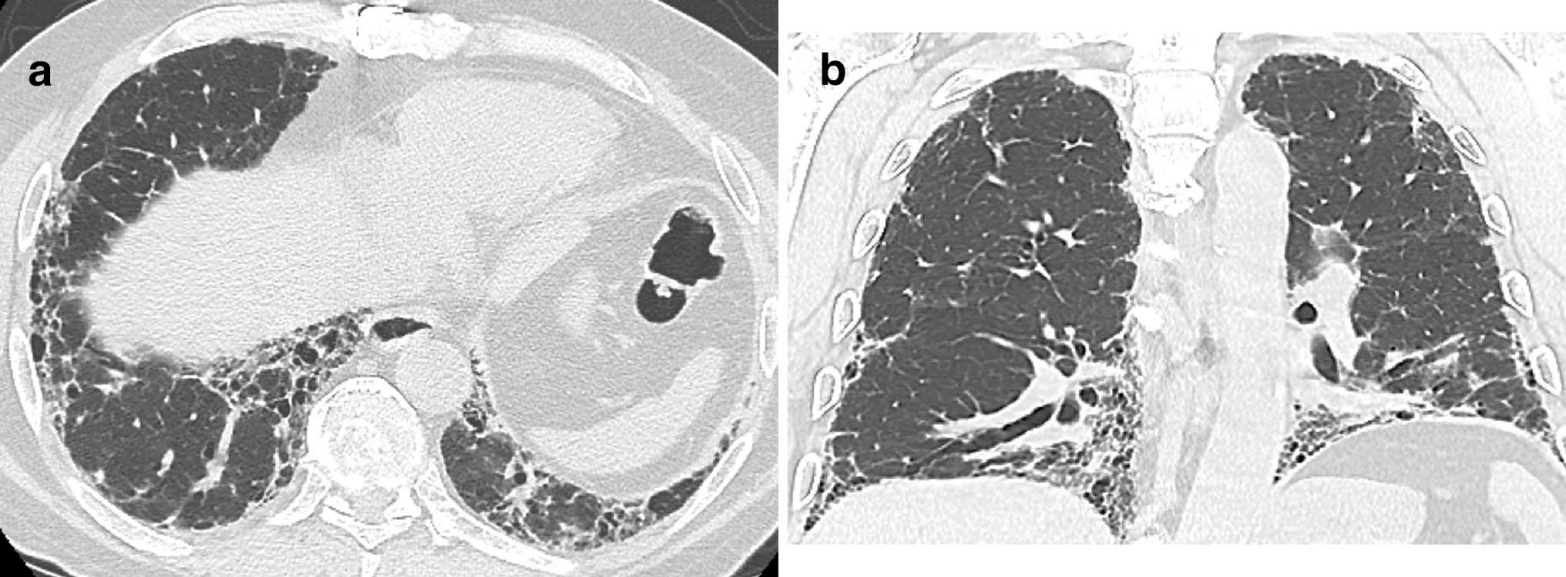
Axial (a) and coronal (b) HRCT images show peripheral and basilar predominant pulmonary fibrosis characterized by reticulation, traction bronchiectasis, traction bronchiolectasis and subpleural honeycombing consistent with UIP
